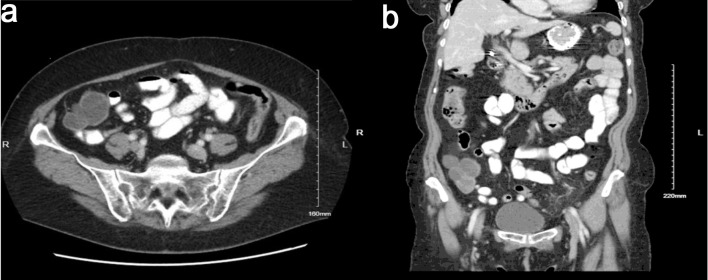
Axial (a) and coronal (b) computed tomography (CT) images demonstrating an omental cystadenofibroma. The lesion appears as a well-circumscribed cystic mass within the anterior abdomen, consistent with a benign omental implant. The coronal view (b) provides a complementary perspective showing the lesion’s relationship to adjacent bowel loops and pelvic organs, further emphasizing its benign, non-infiltrative appearance.
